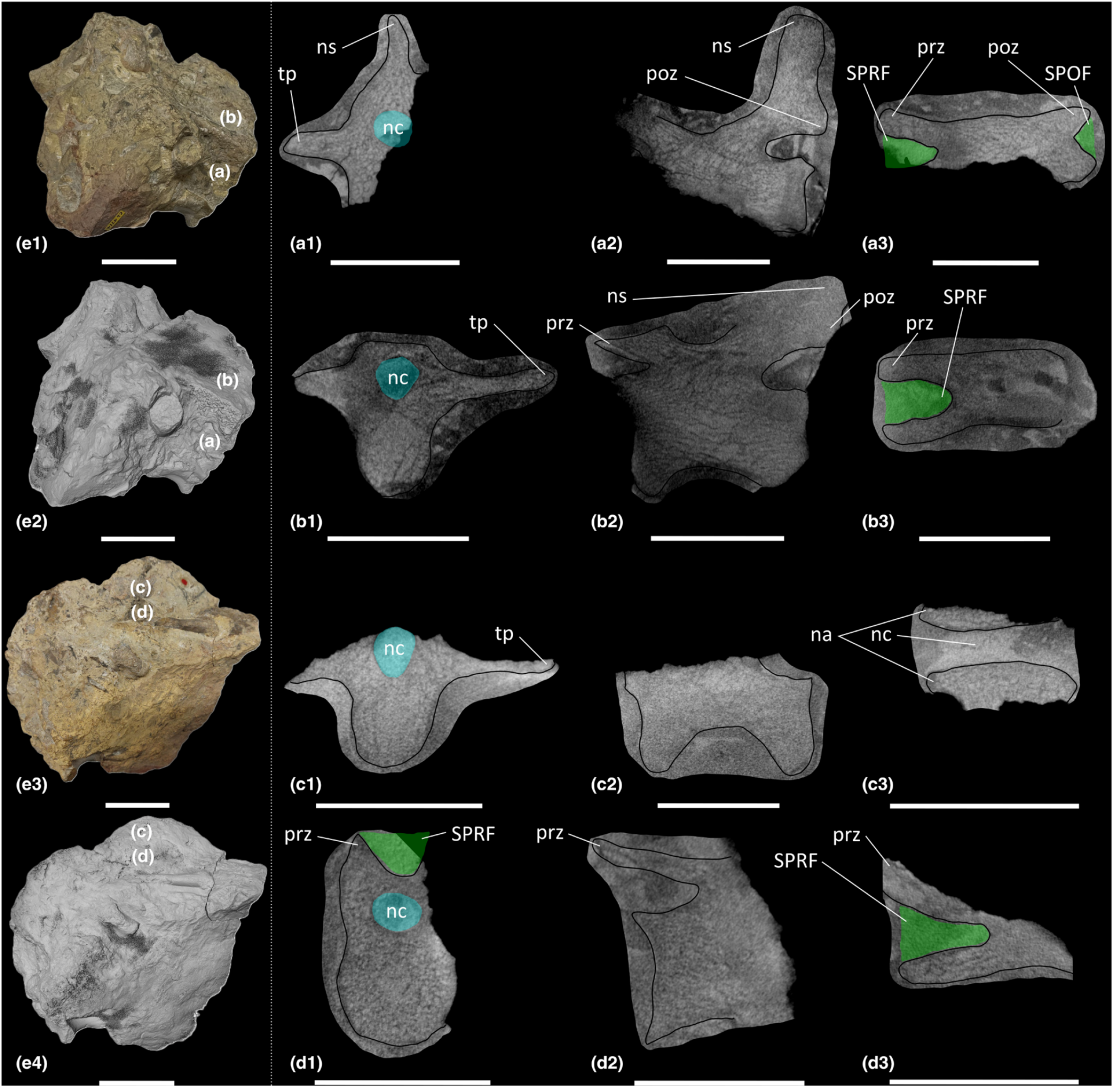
CT scan data of *Thecodontosaurus antiquus* caudal vertebrae in Cb 4714 block. (a) element A, (b) element B, (c) element C, (d) element D; (a1–d1) transverse cross‐section, (a2–d2) parasagittal cross‐section, (a3–d3) horizontal cross‐section; (e1–e2) side 1 of block, (e3–e4) side 2 of block; (e1, e3) photograph of block, (e2, e4) surface scan of block. na, neural arch; nc, neural canal; ns, neural spine; poz, postzygapophysis; prz, prezygapophysis; SPOF, spinopostzygapophyseal fossa; SPRF, spinoprezygapophyseal fossa; tp, transverse process. Key: Blue highlight, neural canal; green highlight, fossa. Scale bars = 2 cm (a–d), 5 cm (e).
